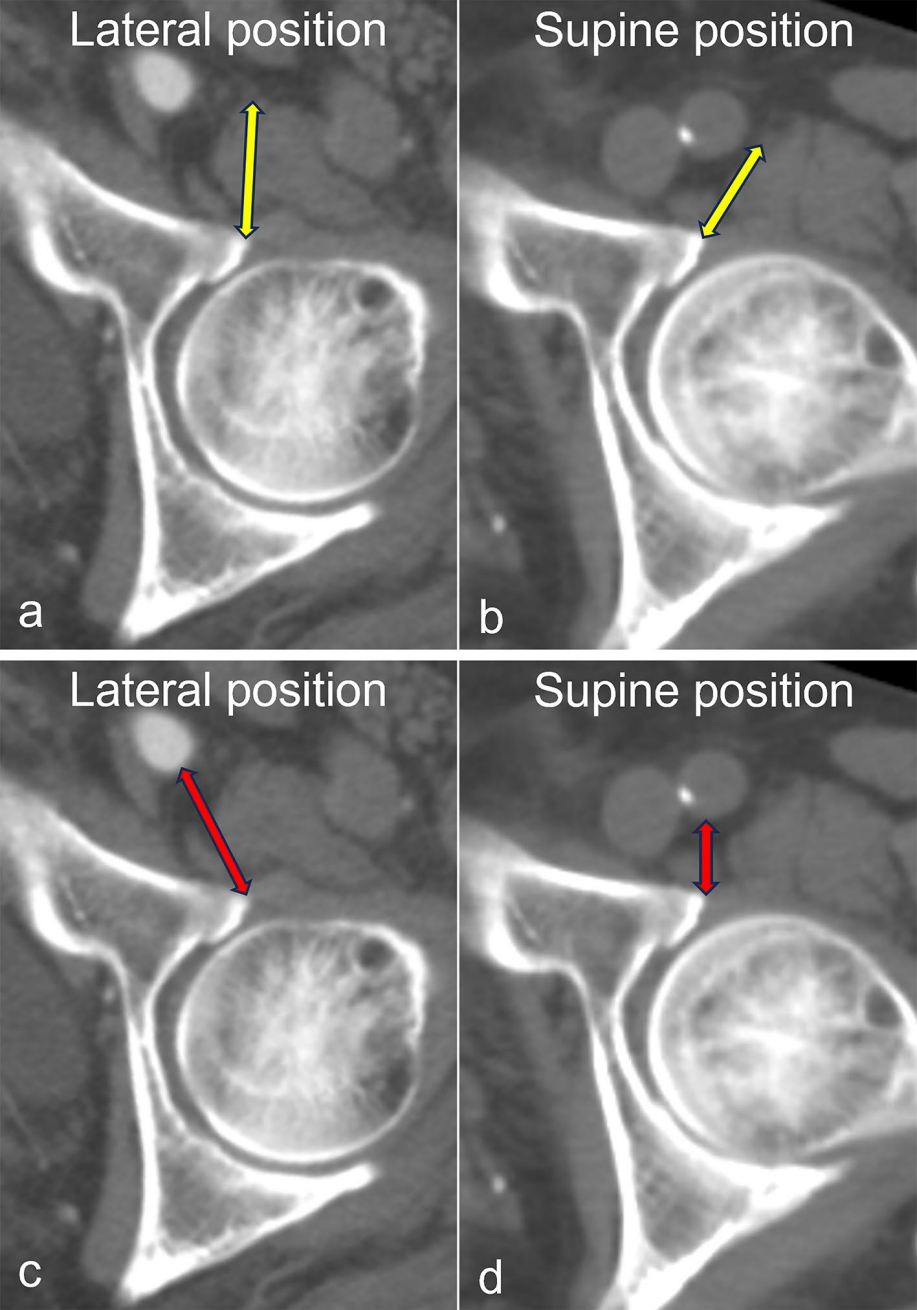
Computed tomography images showing Distances N and A. (a) Distance N in the lateral position. (b) Distance N in the supine position. (c) Distance A in the lateral position. (d) Distance A in the supine position. Distance N, distance from the anterior margin of the acetabulum to the femoral nerve; Distance A, distance from the anterior margin of the acetabulum to the femoral artery
